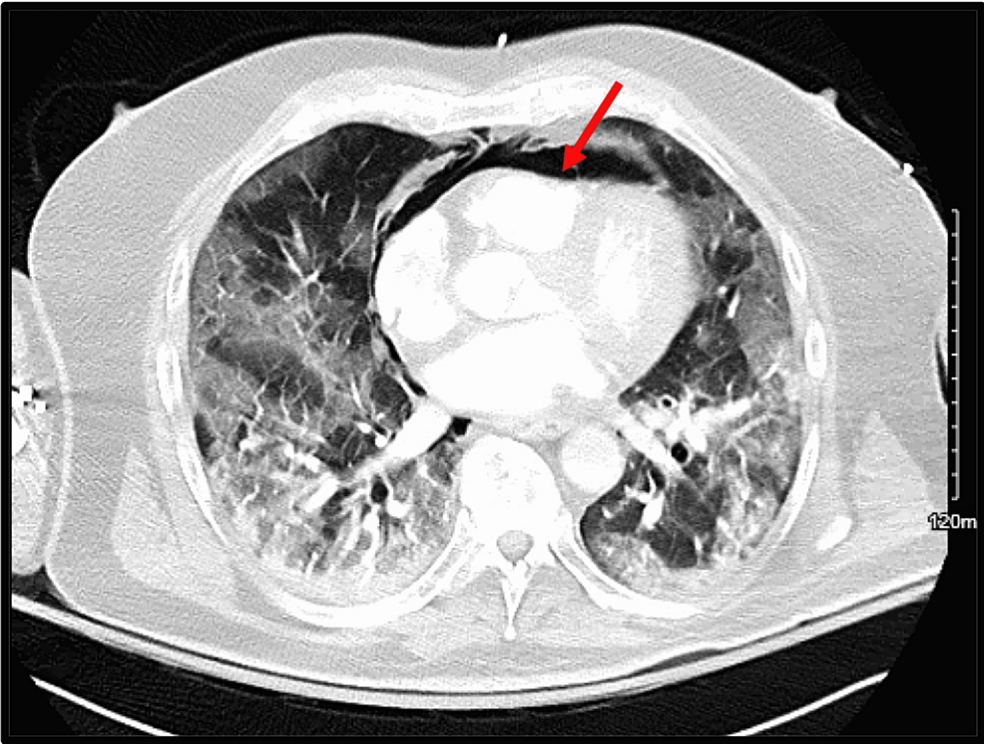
CT chest showing pneumomediastinum (red arrow)
